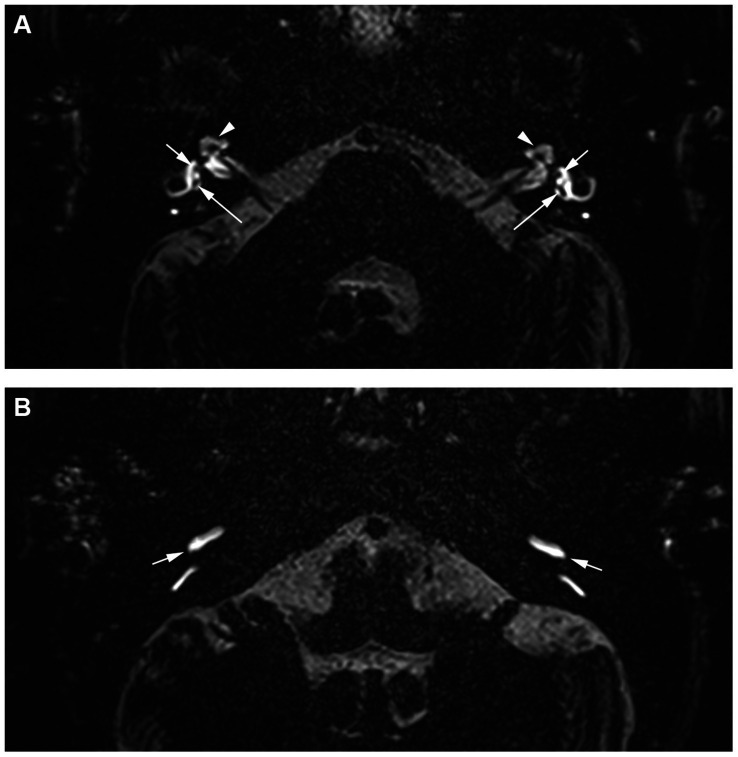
A 66-year-old woman with a history of almost daily short attacks of vertigo lasting up to 10 min, accompanied by nausea. These attacks are followed by pronounced headaches lasting for the rest of the day, accompanied by photophobia and phonophobia. During these attacks, she also experiences pressure in both ears with bilateral tinnitus but no hypoacusis. (A) An axial 4 h-delayed Gd-enhanced 3D SPACE FLAIR sequence at the level of the vestibule and the apical turn of the cochlea. There is a normal slit-like appearance of the scala media in the apical turn of the cochlea on both sides (arrowhead). A normal, small, and separately visible saccule is located anterior and medial in the vestibule on both sides (small arrow), and a larger utricle is positioned more posterior and lateral (large arrows). (B) An axial 4 h-delayed Gd-enhanced 3D SPACE FLAIR sequence of the basal turn of the cochlea and the posterior semicircular canal shows symmetrical PLE in the basal turn of the cochlea. In conclusion, the absence of CEH, VEH, and asymmetrical PLE supports the diagnosis of VM.
